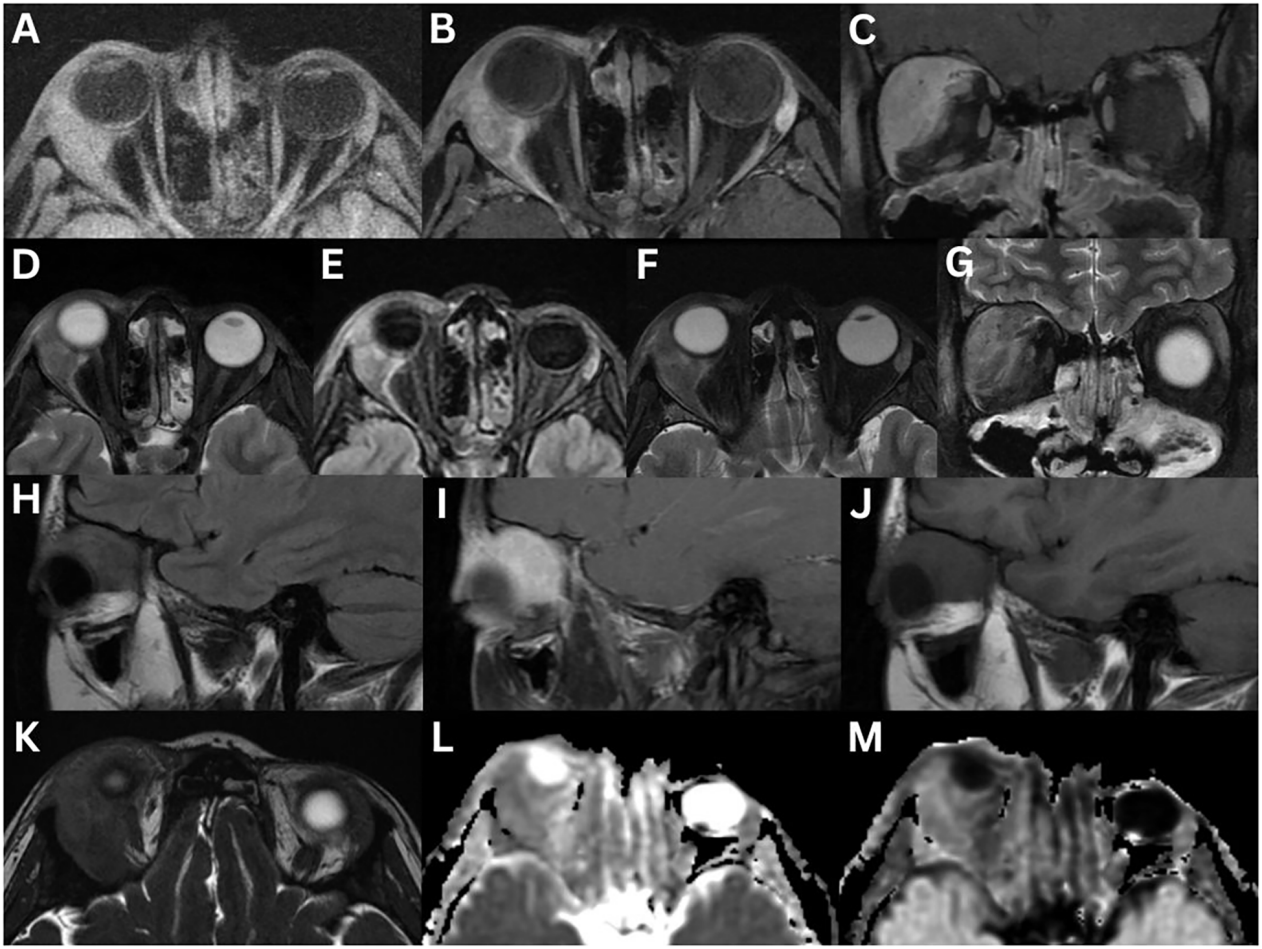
Eosinophilic Granulomatosis with Polyangiitis with involvement of the right lacrimal gland. MRI T1-weighted axial fat-suppressed FLAIR (A) and fat-suppressed contrast-enhanced axial (B) and coronal (C), T2-weighted axial (D), axial fat-suppressed FLAIR (E), axial fat-suppressed (F) and coronal fat-suppressed (G), T1-weighted sagittal FLAIR (H), and sagittal fat-suppressed contrast-enhanced (I), and T2-weighted sagittal FLAIR (J) sequences demonstrate an enlarged right lacrimal gland with an ill-defined outline and heterogeneous contrast enhancement. The lesion encases the adjacent superior rectus complex and right lateral rectus muscle, as well as the adjacent orbit displacing them inferomedially. Axial 3D Fiesta is also shown (K). There is mild diffusion restriction on axial ADC (L) and axial eADC (M).
